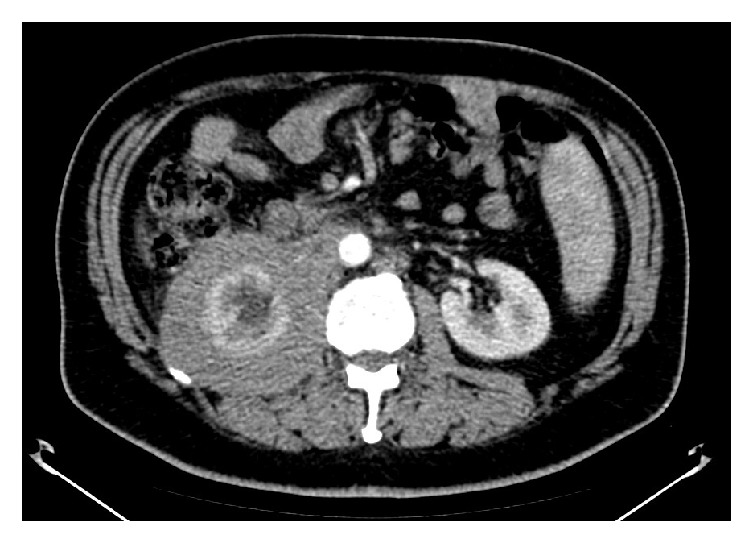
Preoperative computed tomography (CT) scan showing a right renal mass.
